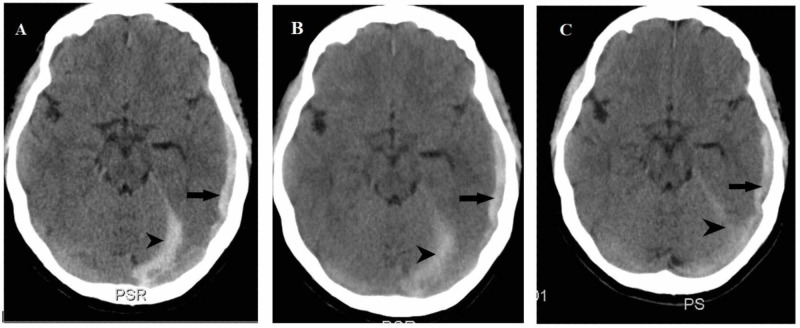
Non-contrast axial computed tomography brain (A) on readmission showing left temporal (black arrow) and left tentorium-cerebelli (arrowhead) subdural hemorrhage with stable course on day two (B) and decreased hemorrhage size of tentorium-cerebelli on day five (C)
